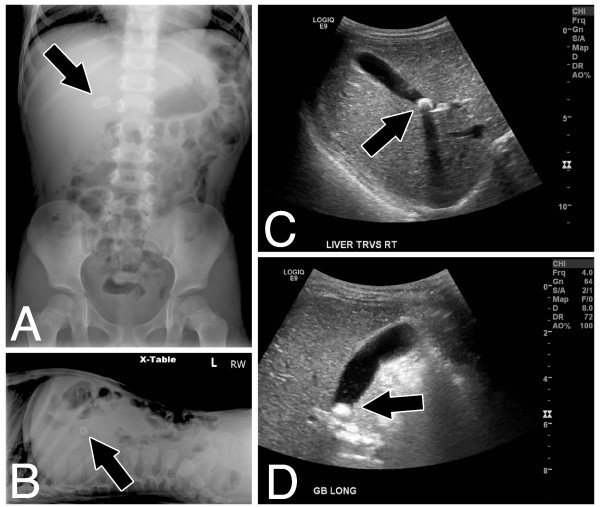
Panel A: anterior/posterior abdominal radiograph. Panel B: Cross-table abdominal radiograph. Panels C, D: Transverse and longitudinal ultrasound views of liver and gallbladder. Arrows indicate the gallstone.
